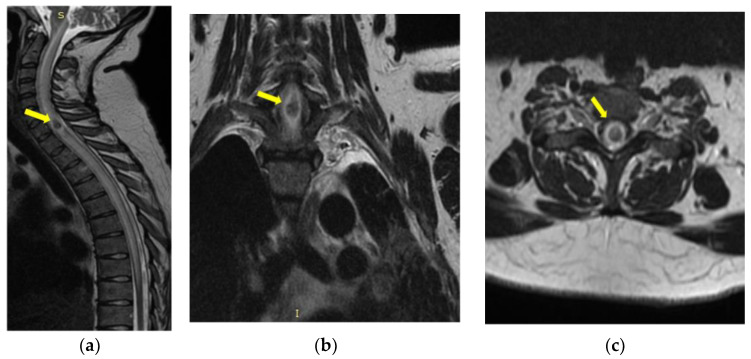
MRI of the cervical spine T2-weighted image—sagittal (a), coronal (b), and axial (c) sections: cord expansion with a decreased signal from abscess core and increased signal from surrounding edema. The yellow arrow indicates the expansive cystic intramedullary process.
